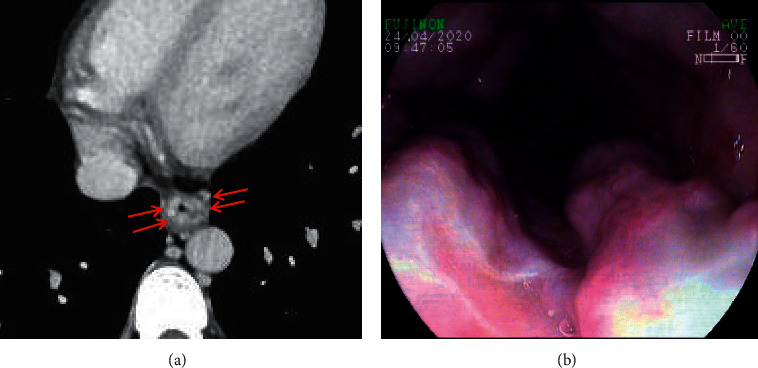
Esophageal varices. In a 38-year-old male with liver cirrhosis, the axial contrast-enhanced CT scan (a) on the portal vein phase demonstrated that esophageal varices (red arrow) were enhanced channels adjacent to the surface of the esophageal lumen, with round, tubular, or scalloped borders. Upper gastrointestinal endoscopy (b) showed several tortuous varices in the esophagus.
